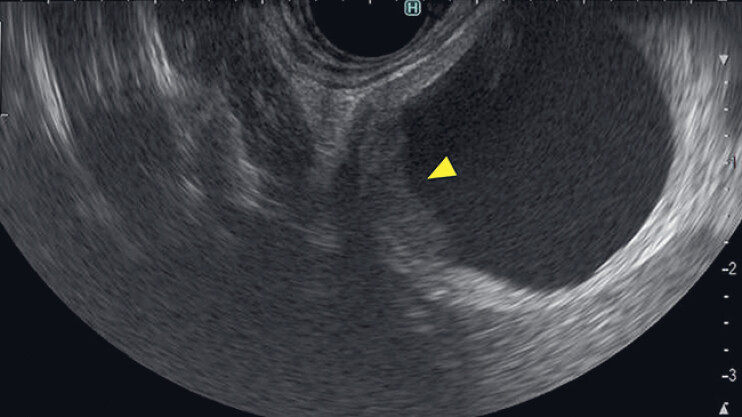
Endoscopic ultrasound shows a hypoechoic thickened wall in the cystic lesion.
